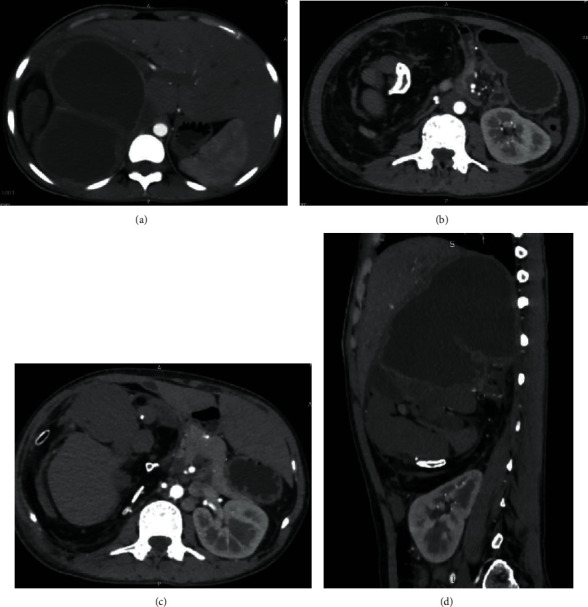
Abdominal computed tomography shows a large heterogeneous retroperitoneal mass with cystic, calcified, soft tissue and lipomatous components (a). Large cystic component (b). Well-formed vertebral body with surrounding lipomatous tissue (c). Well-formed ribs and long bone (d). Inferior displacement of the right kidney by mass.
